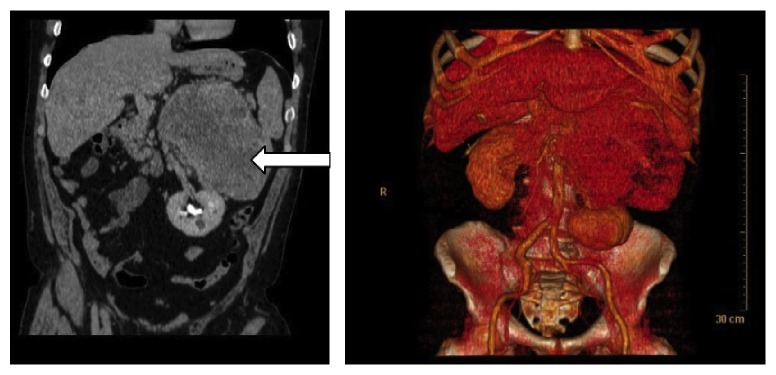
Adrenal CT Image shows a tumor on the left adrenal gland of 202 × 127 × 202 mm (white arrow) that displaces ipsilateral kidney and renal cysts.
